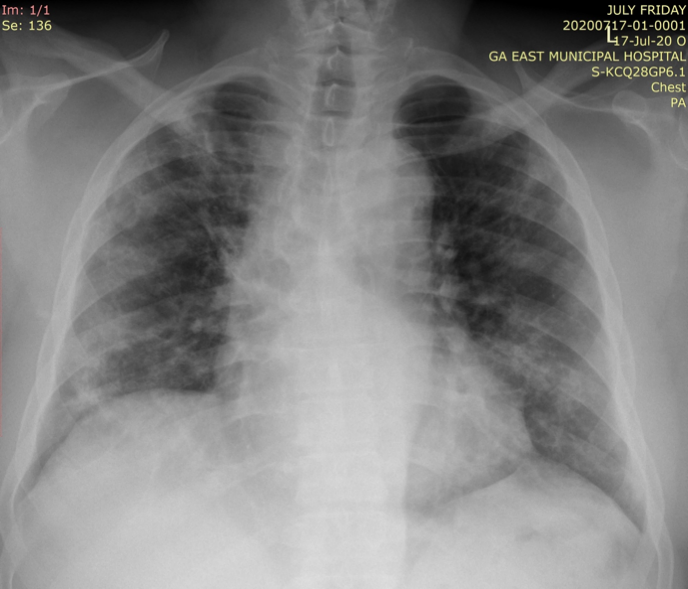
Posteroanterior chest radiograph showing bilateral ground glass opacities in all the zones of both lung fields - Moderate to Severe Disease. Also noted are elevated right hemidiaphragm, tenting of the left hemidiaphragm and unfolding of the aorta
